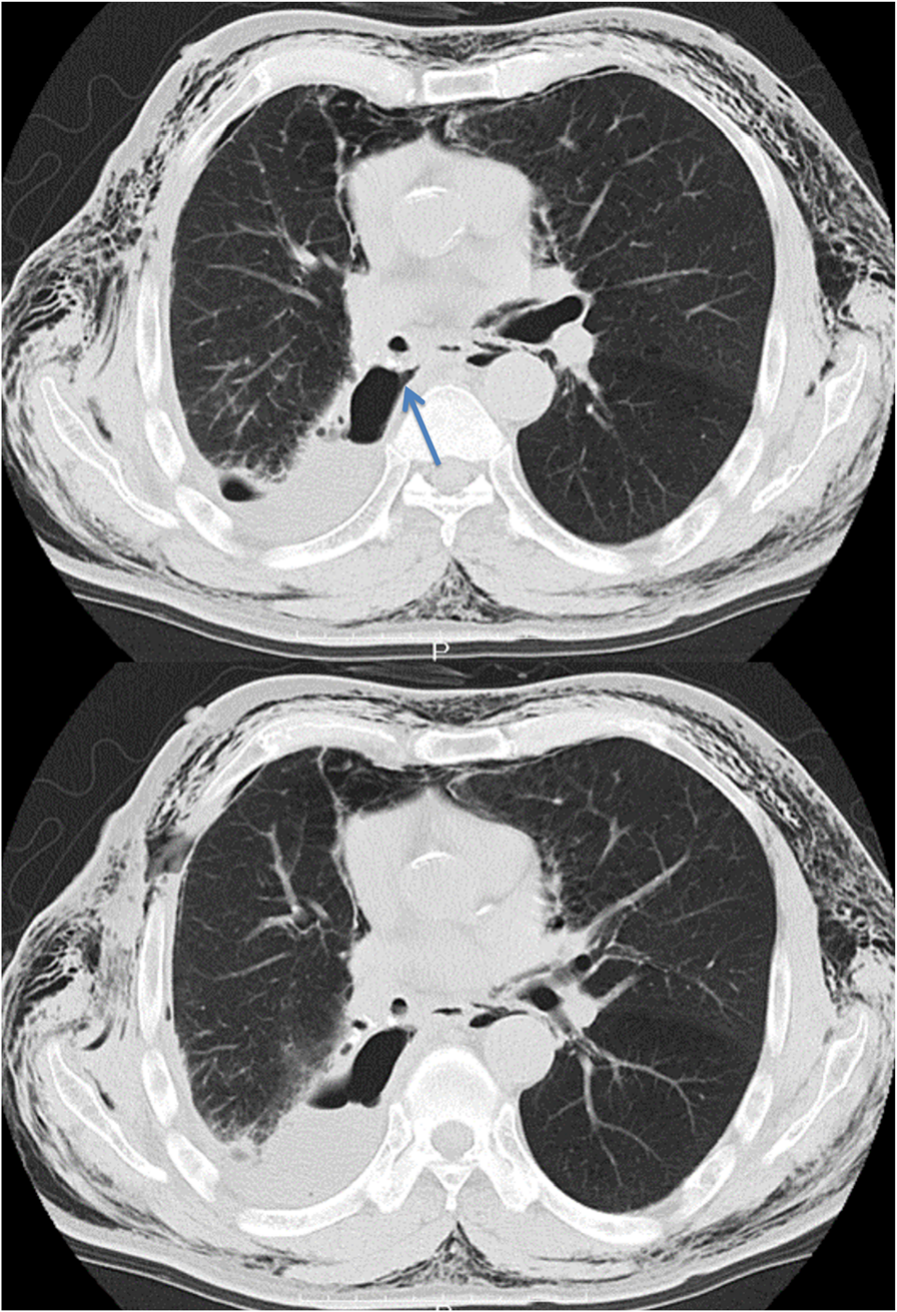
CT image before re-operation, on POD 12 following the primary operation (i.e., right lower lobectomy). The arrow shows the broken appearance of the bronchial stump. CT computed tomography, POD postoperative day
